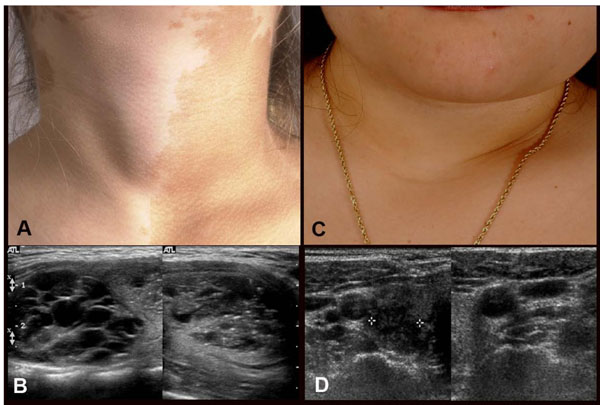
Clinical and ultrasound findings of thyroid involvement in FD/MAS. Panels A & B demonstrate the findings in a 9-year-old girl with MAS and hyperthyroidism. A goiter is clearly seen on inspection (A) and the ultrasound (B) shows the typical cystic (Swiss cheese) appearance that seen in MAS thyroids. Panels C & D demonstrate the findings of a 30-year-old woman with MAS. While no goiter was evident on inspection, nor was one obvious on palpation, the ultrasound clearly demonstrated the typical findings seen in ultrasounds of patients with MAS and thyroid involvement. Adapted from reference [13.]
